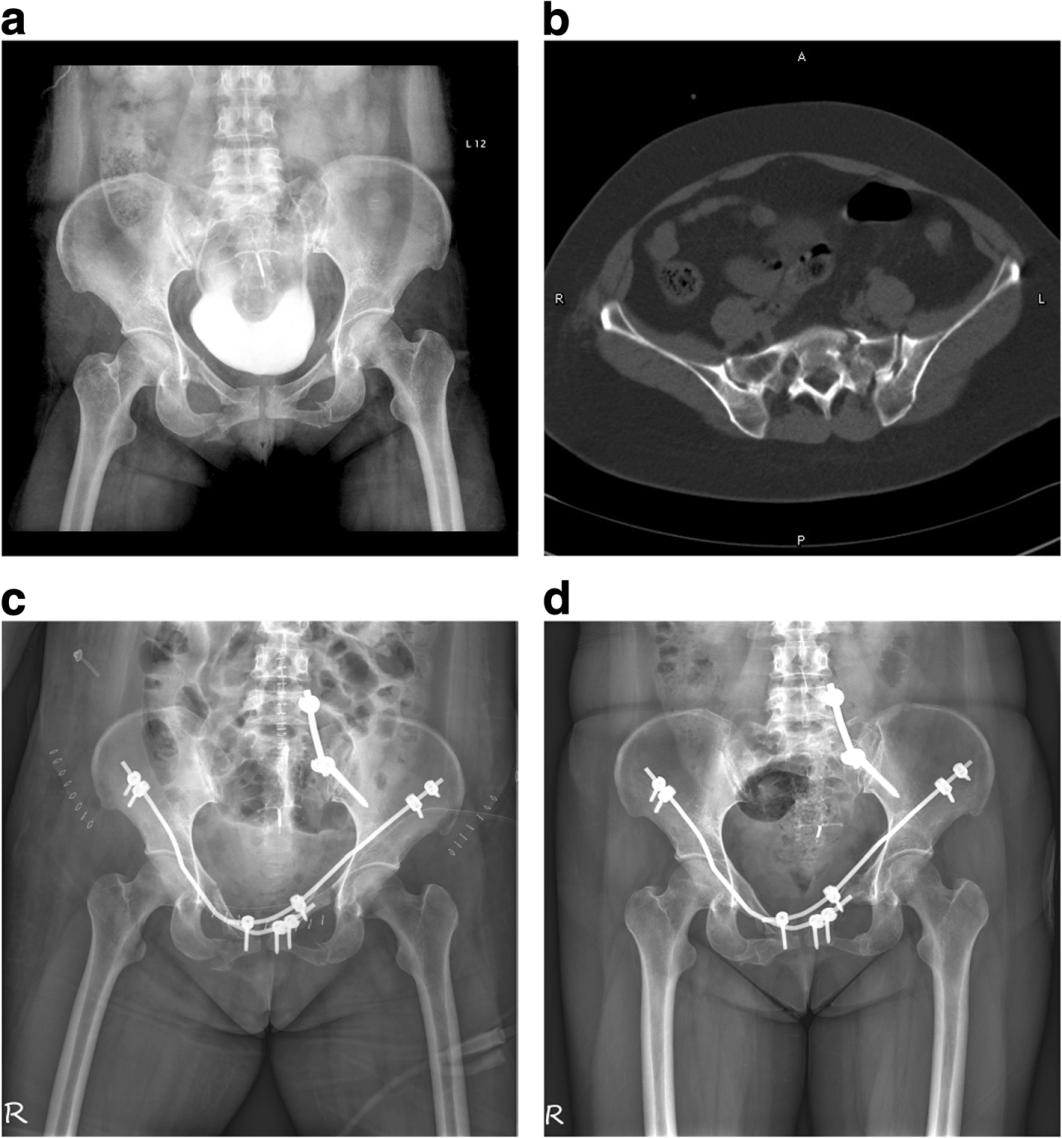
A 44-year-old woman with anterior and posterior pelvic ring injuries caused by a fall. a Preoperative radiology plain images showing bilateral pubic rami fracture. b Preoperative axial computed tomography scan image showing a left side sacral fracture. c Postoperative radiology plain images showing good reduction with the modified pedicle screw-rod fixation technique for the anterior ring and spinal pelvic fixation for the sacral fracture. d Radiology plain image showing fracture healing at 8 months postoperatively
